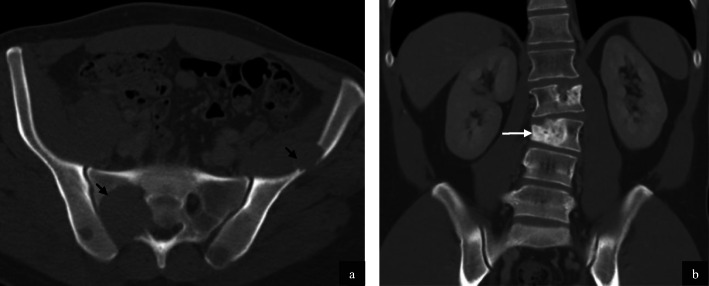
Bone metastases in two patients with alveolar soft part sarcoma. Axial CT of the pelvis on bone windows demonstrates multiple lytic metastases in this 69 year old male patient (a, black arrows). Coronal CT of the abdomen on bone windows in a 31 year old male patient demonstrates mixed sclerotic/lytic metastasis (b, white arrow) with associated with vertebral body collapse
